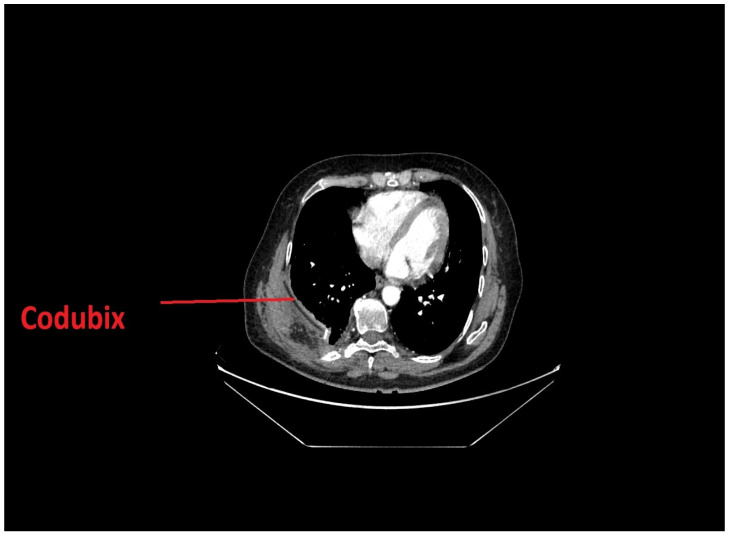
Chest wall CT scan after prosthesis implantation.
